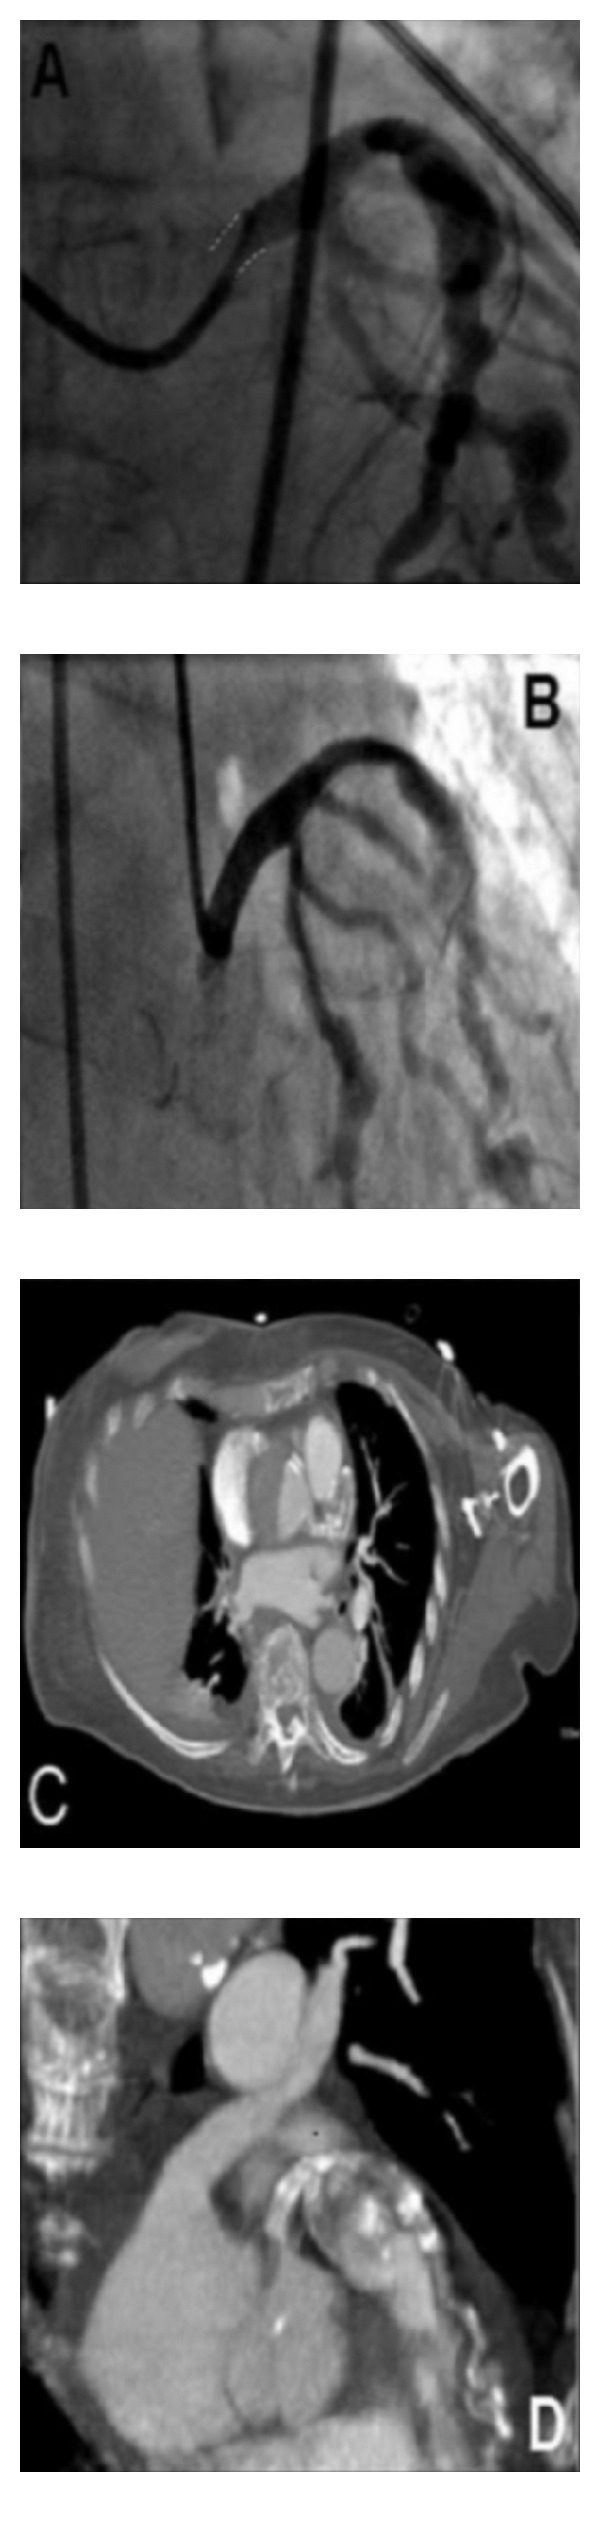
Angiographic projections in (A) and (B); (A) (35°LAO and 24°CRA): significant length reduction (dashed lines) of the LMCA; (B) (28°RAO and 6°CAU): PCI with implantation of 4.5 × 13 mm BMS on the proximal tract of the LMCA overlapping with the previously implanted stent. CT scan in (C) (axial scanning) and (D) (3D volume rendering reconstruction) whereby aortic dissection involving the LMCA ostium and thrombosis of the coronary aneurism are seen. LAO: left anterior oblique; RAO: right anterior oblique; CRA: cranial; CAU: caudal.
